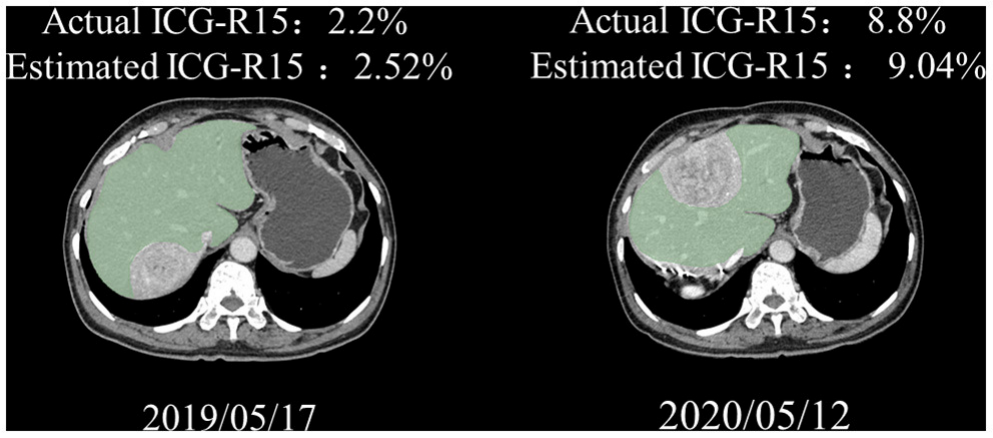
Representative paired CT images of a 68-year-old man. Great agreement was observed between actual and estimated ICG-R15. ICG-R15, indocyanine green retention rate at 15 min; CT, computed tomograph.
